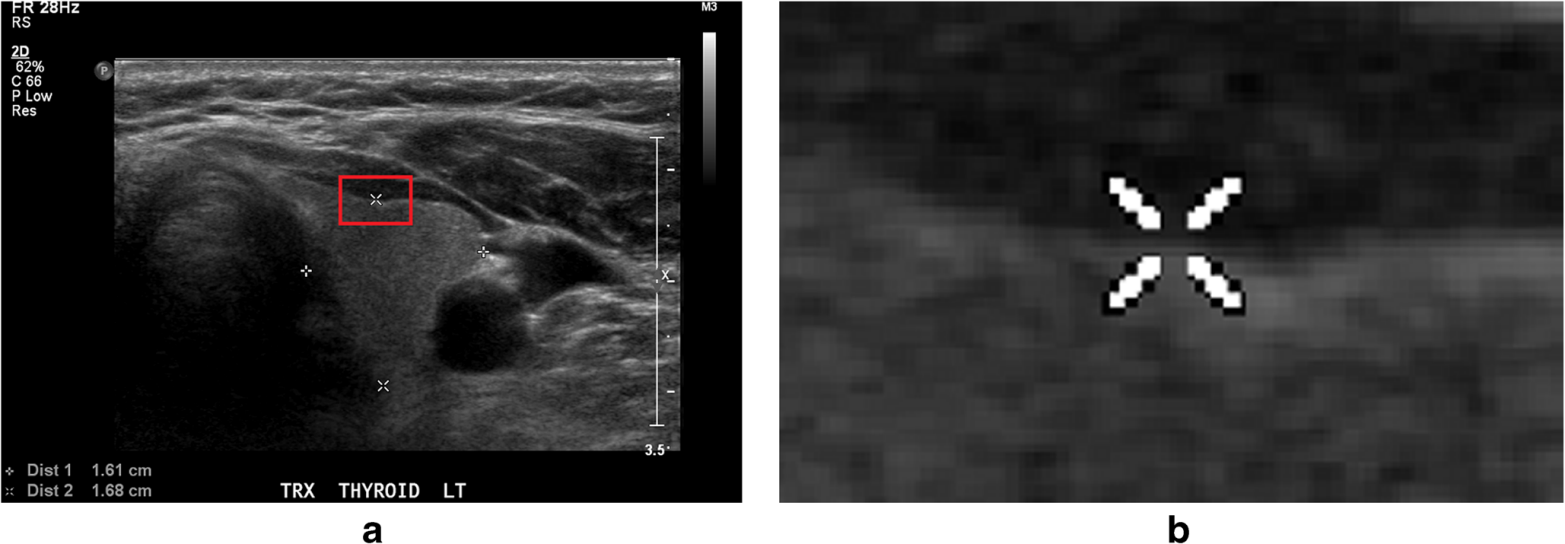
Example of artifacts made by radiologist on the thyroid ultrasound image. a Ultrasound image with artifacts covering the textures. b Details of how the artifact covers the ultrasound textures in the image, bounded by the *red rectangle* in a
